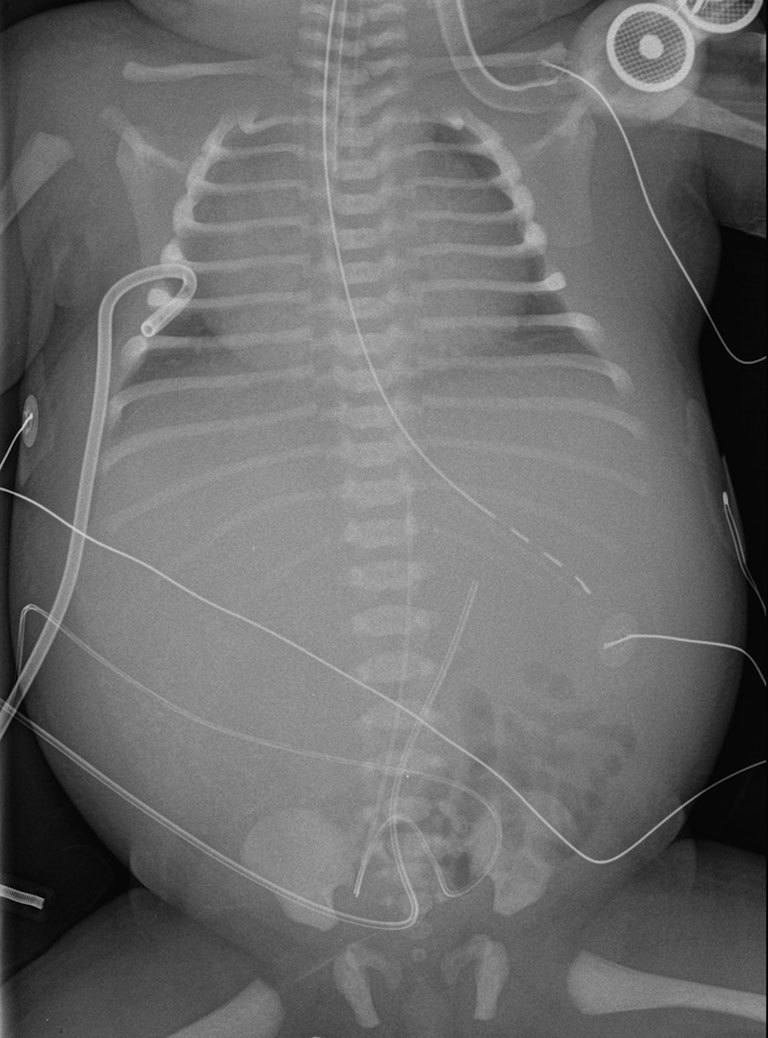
X-ray of the chest and abdomen showed cardiomegaly, acute pulmonary and soft tissues oedema, and an opacity related to hepatomegaly.
